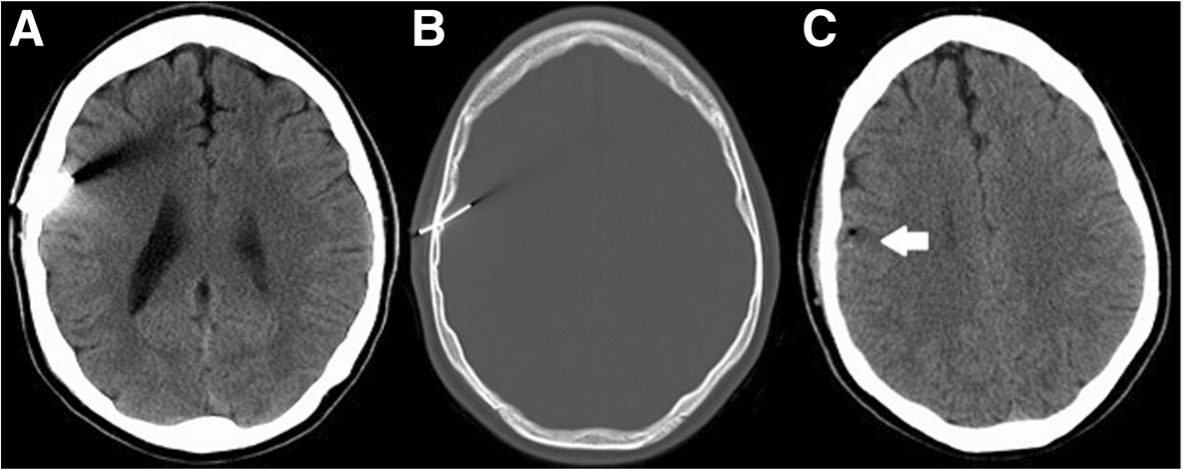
Computed tomography of the patient’s head without contrast. a Brain window. b Bone window. A metallic nail in the right parietal bone and parietal lobe in the preoperative study which is removed in the postoperative study (c) with small air pocket and extra-axial hematoma in the same level
